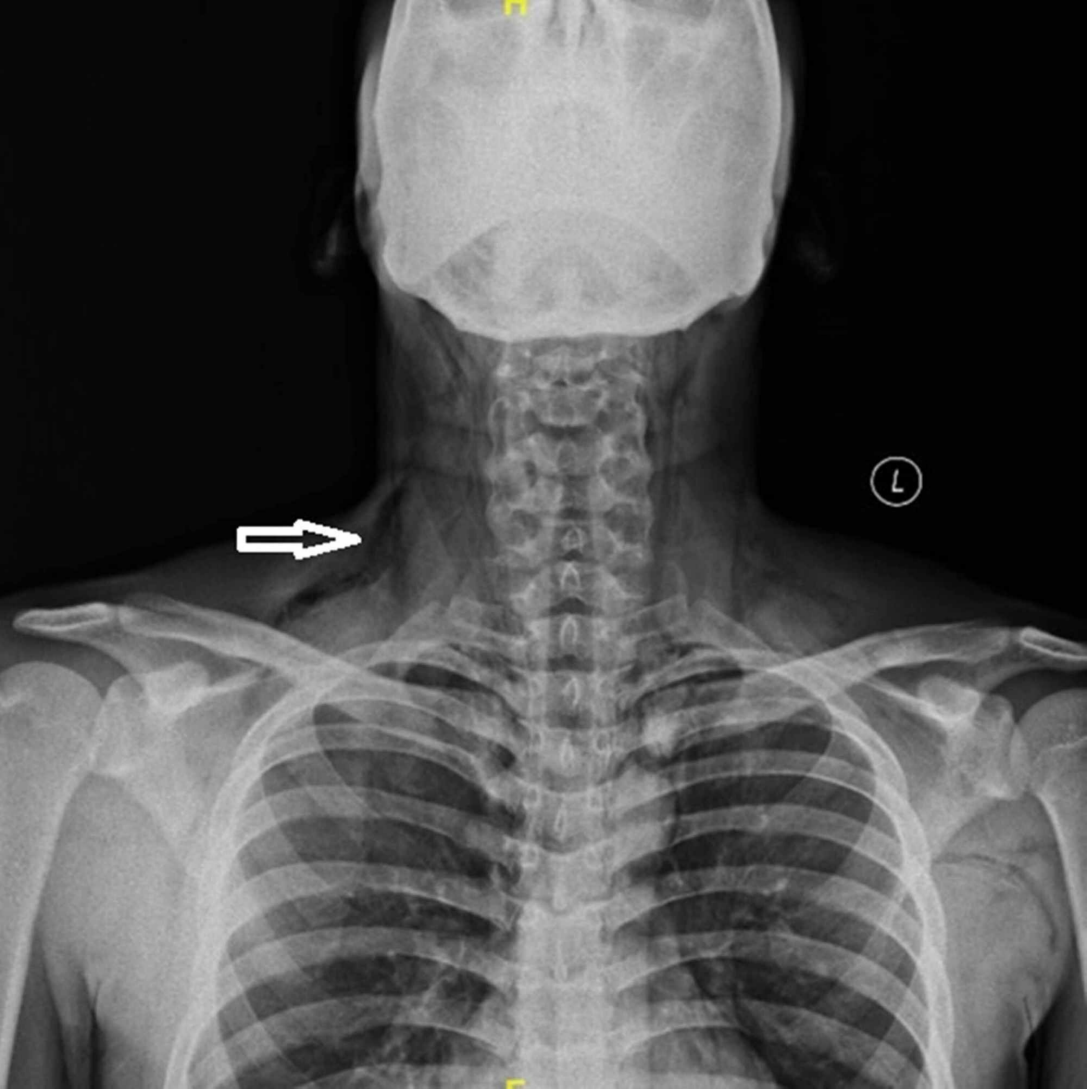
X-ray neck showing subcutaneous emphysema (arrow)
